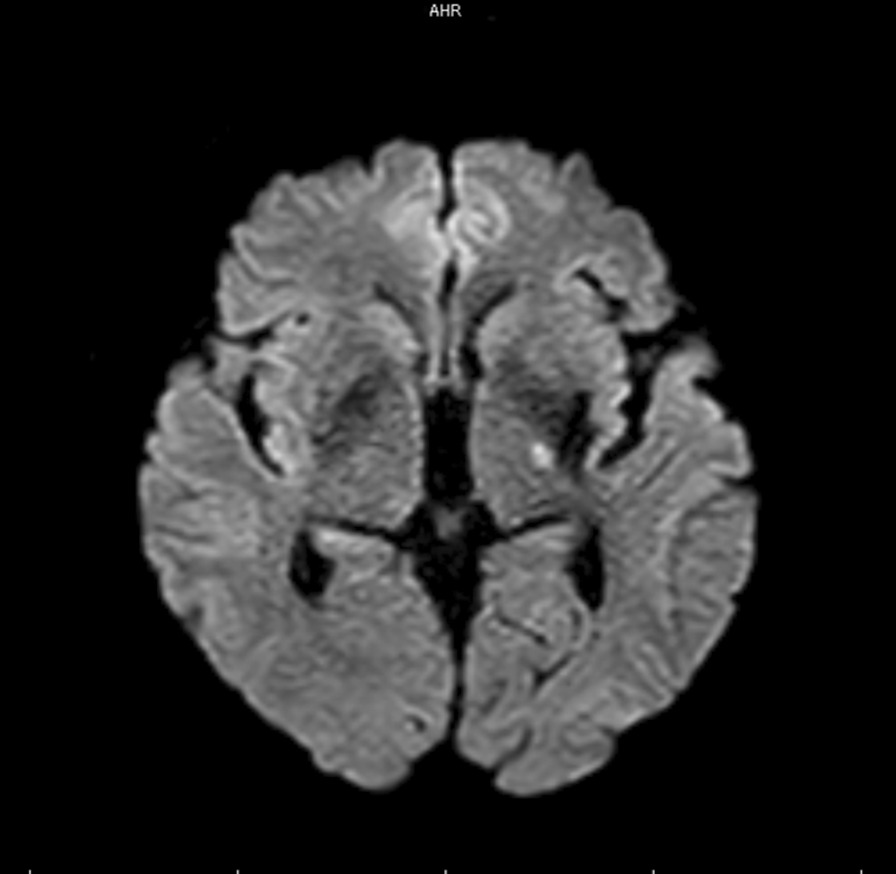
Head CT immediately after onset. The diffusion-weight images of the head showing a lesion of the left posterior limb of the internal capsule reported as a left entropion perforator branch infarction.
